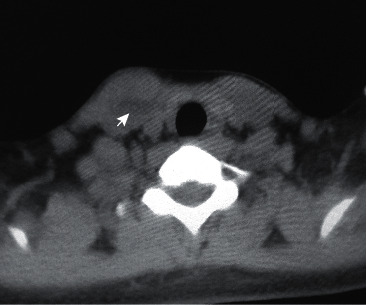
Axial noncontrast computed tomography image of the neck at the level of T1 vertebra showing slightly hypodense cystic lesion with a small area of hyperdensity within the lesion (arrow).
